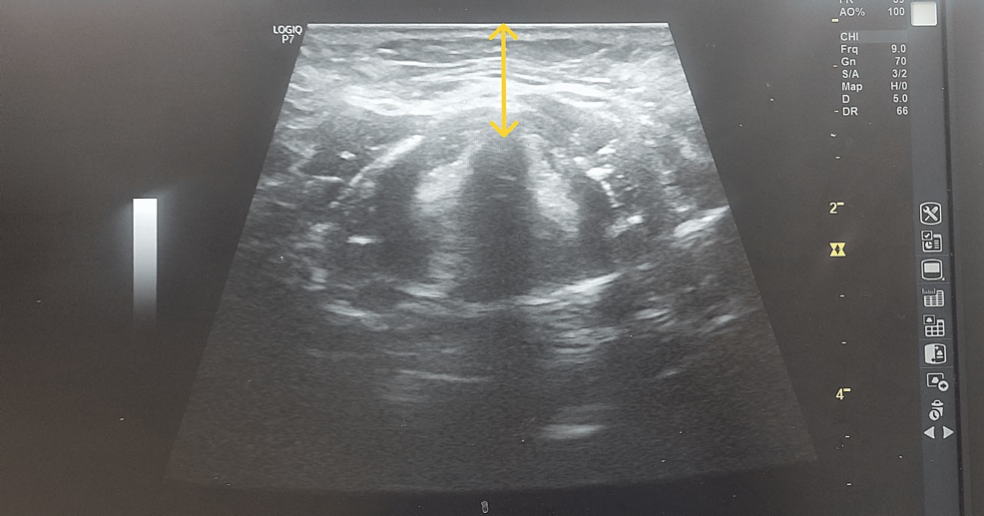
Ultrasound measurement of anterior neck soft tissue thickness at the level of vocal cord.
